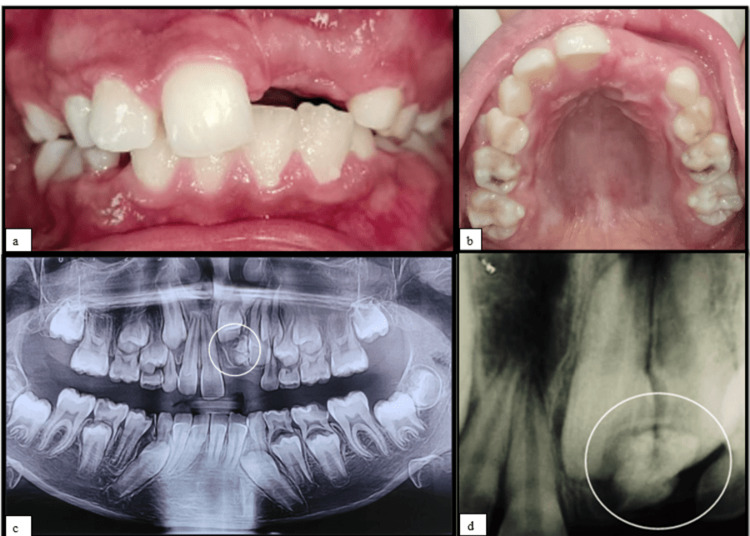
(a, b) Intraoral preoperative pictures showing missing left maxillary permanent central incisor. (c) Orthopantomogram showing large radiopaque calcified mass wrt 21,22. (d) Intraoral periapical radiograph showing large radiopaque mass with radiodensity as that of a tooth wrt impacted 21,22 21: left maxillary permanent central incisor; 22: left maxillary permanent lateral incisor; wrt: with respect to
